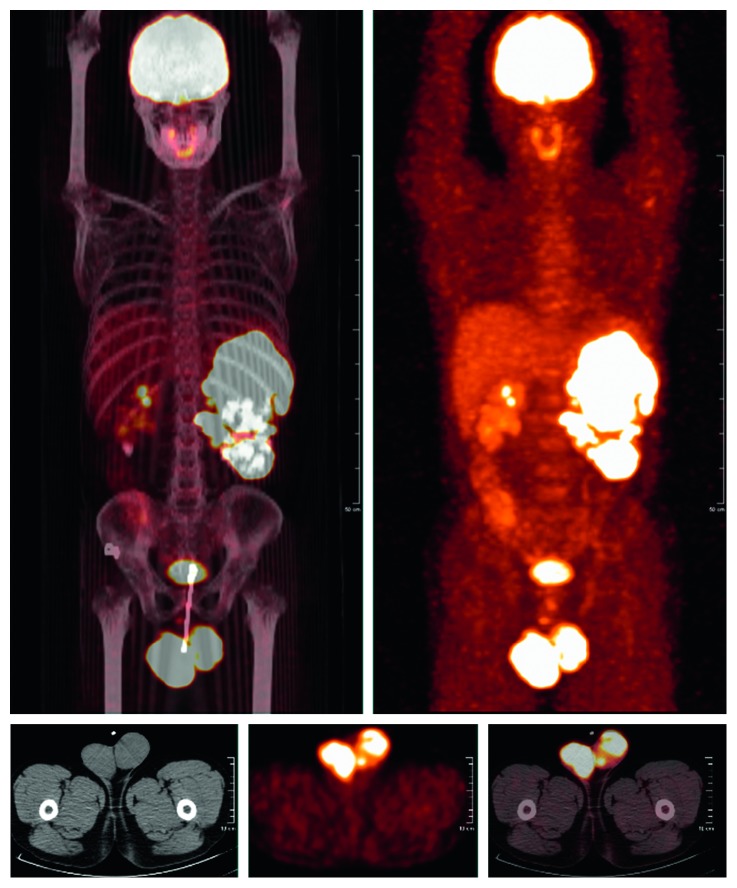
A 37-year-old patient was diagnosed with diffuse large B-cell lymphoma that involved with bilateral testes. The PET/CT showed asymmetrical increased uptake in the bilateral testes. He received orchiectomy, prophylactic intrathecal injection, and 6 cycles of chemotherapy with rituximab-etoposide, prednisone, oncovin (vincristine), cyclophosphamide, and hydroxydaunorubicin (doxorubicin) (R-DA-EPOCH). The duration from the time of diagnosis to the date when radiological findings suggested suspected pancreatic involvement was 9.3 months. The patient was alive till October 30, 2017, after a follow-up of 26 months.
